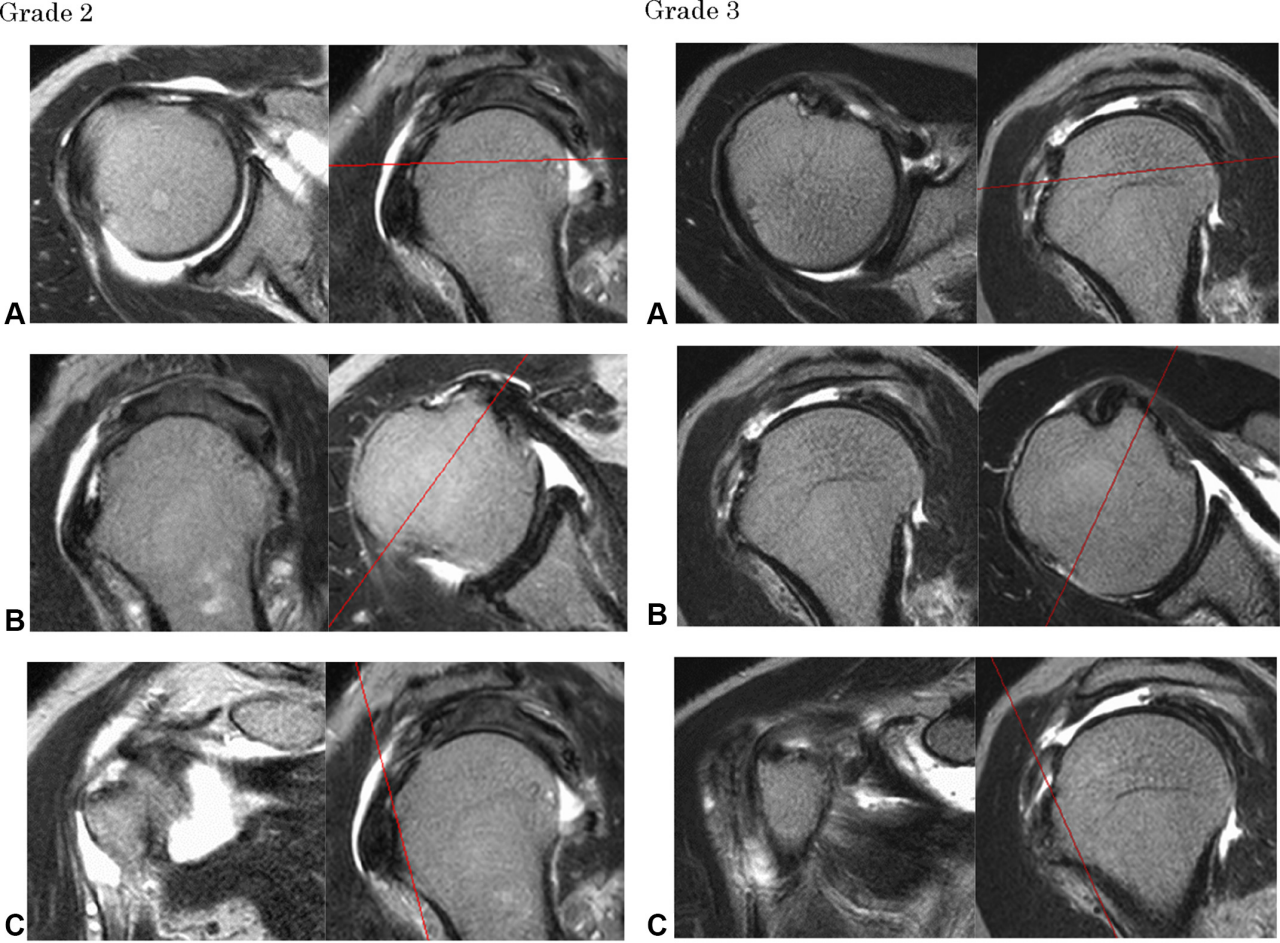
cMRI images of the classification of the anterosuperior aspect STT. Grade 0, intact; grade 1, slight thinning or high intensity of the SSC; grade 2, considerable thinning of the SSC; and grade 3, complete tear. (A) Axial view of cMRI; (B) Oblique sagittal view of cMRI; (C) Oblique coronal view of cMRI. *cMRI*, conventional magnetic resonance imaging; *STT*, subscapularis tendon tear; *SSC*, subscapularis tendon.
